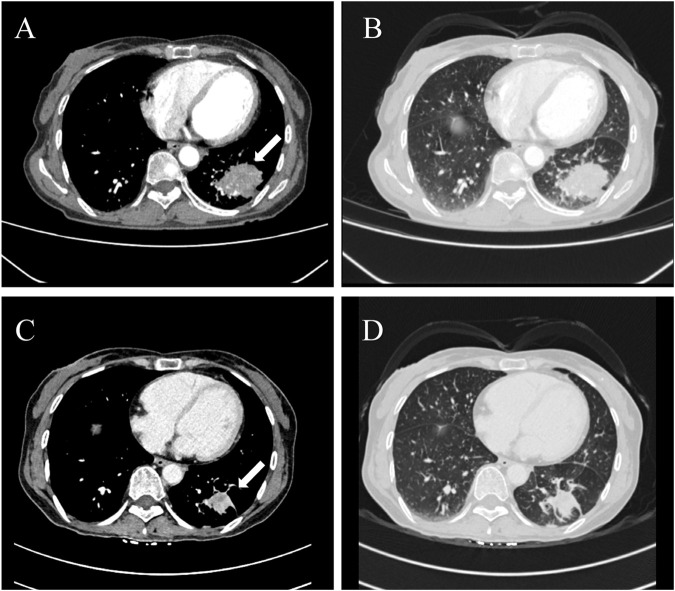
Chest CT evaluation demonstrating the therapeutic efficacy of furmonertinib on the primary lung tumor. (A,B) Baseline CT scans obtained on May 26, 2025. The mediastinal window (A) and lung window (B) reveal a large, solid mass in the left lower lobe (white arrow) prior to treatment. (C,D) Follow-up CT scans obtained on August 2, 2025, coinciding with the onset of hepatotoxicity. The mediastinal window (C) and lung window (D) demonstrate a marked reduction in tumor size (white arrow), indicating a PR to furmonertinib despite the adverse hepatic event.
